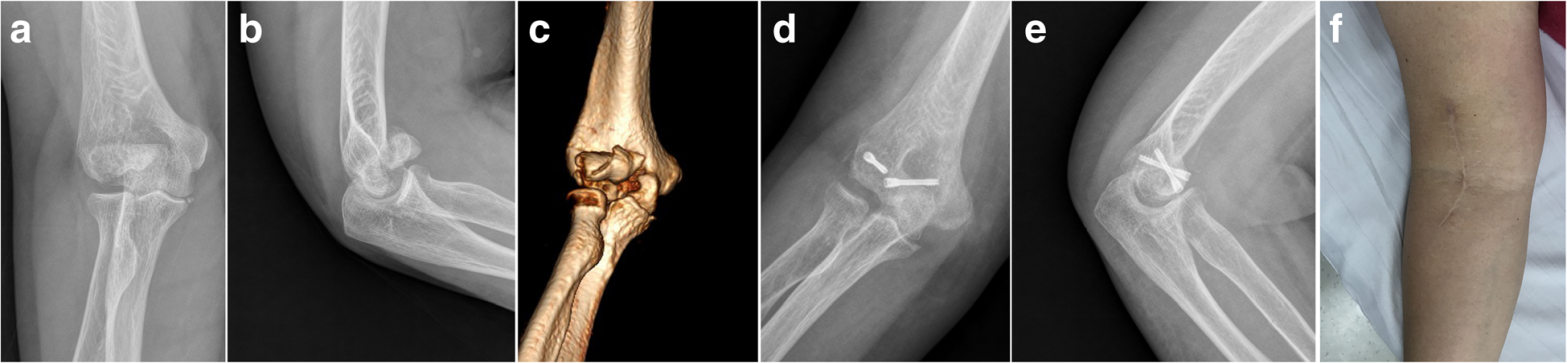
A 64–year-old female with type 3A capitellar fracture surgically treated with open reduction and Herbert screw fixation through anterolateral approach. a and b: Anteroposterior and lateral x-ray views of the fracture preoperatively. c: 3D CT reconstruction of the fracture preoperatively. The fragments were displaced anteriorly and superiorly. d and e: Anteroposterior and lateral x-ray views 12 months postoperatively showed union of the capitellar fracture which was fixed with 2 Herbert screws in an anterior to posterior fashion. f: Incision appearance of anterolateral approach
